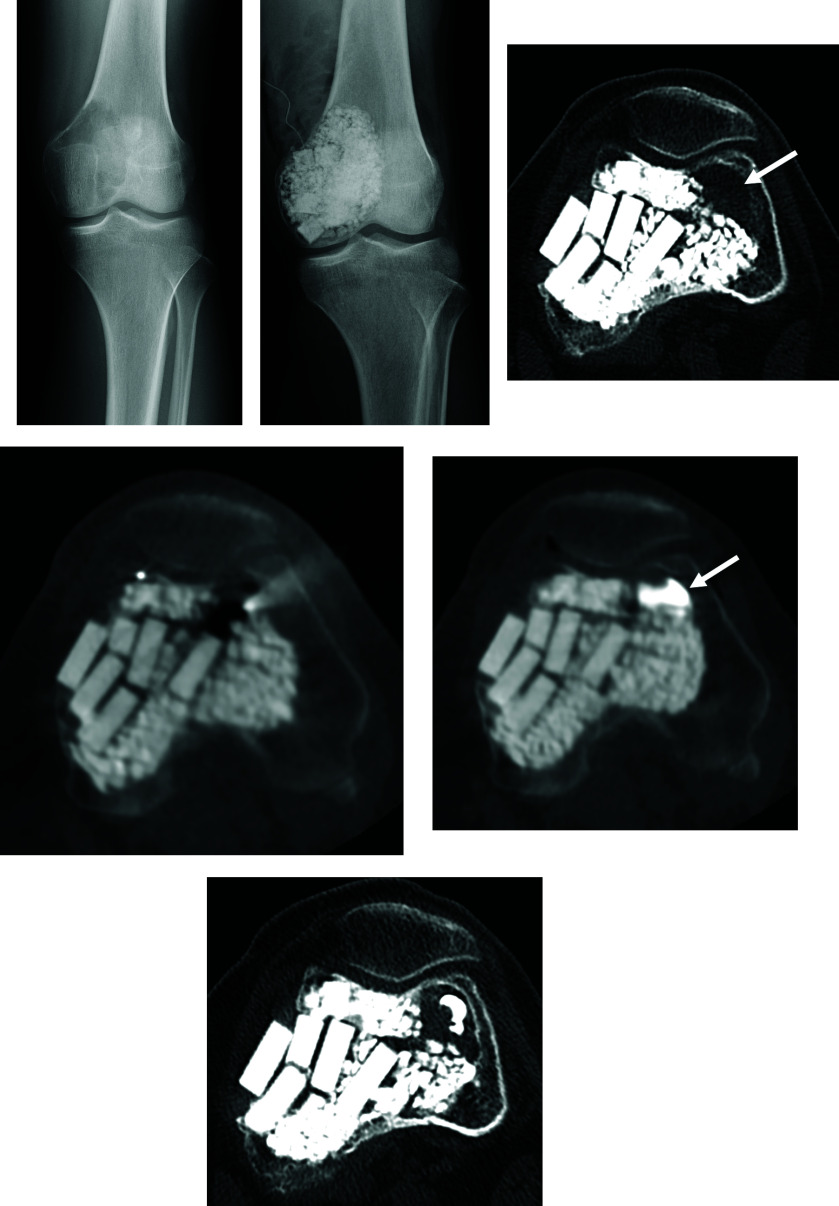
A, Radiography at presentation. B, Radiography after curettage and hydroxyapatite filling. C, CT showed the third local recurrence. After RFA (D), bone cement injection was performed (arrow) (E). F, CT 1 year after RFA showed no local recurrence.
